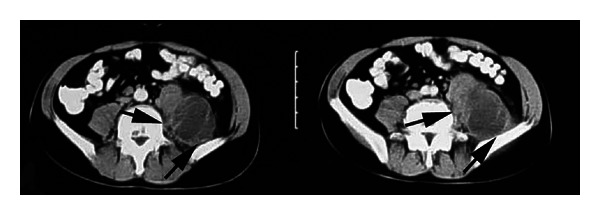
Axial, contrast-enhanced abdominal computed tomography (CT) scans showed huge abscess in a left psoas muscle (arrows) in a patient with “unmasking” tuberculosis- (TB-) associated immune reconstruction inflammatory syndrome (TB-IRIS) (Case no. 2).
